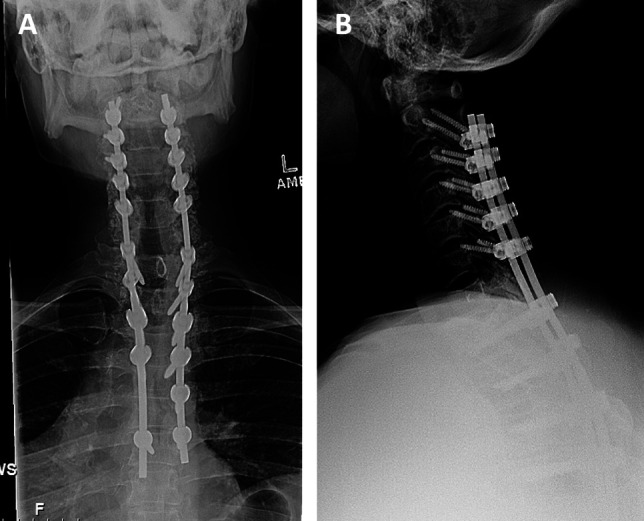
Postoperative standing upright plain radiograph showing cervicothoracic spine AP (A) and lateral (B) views.
